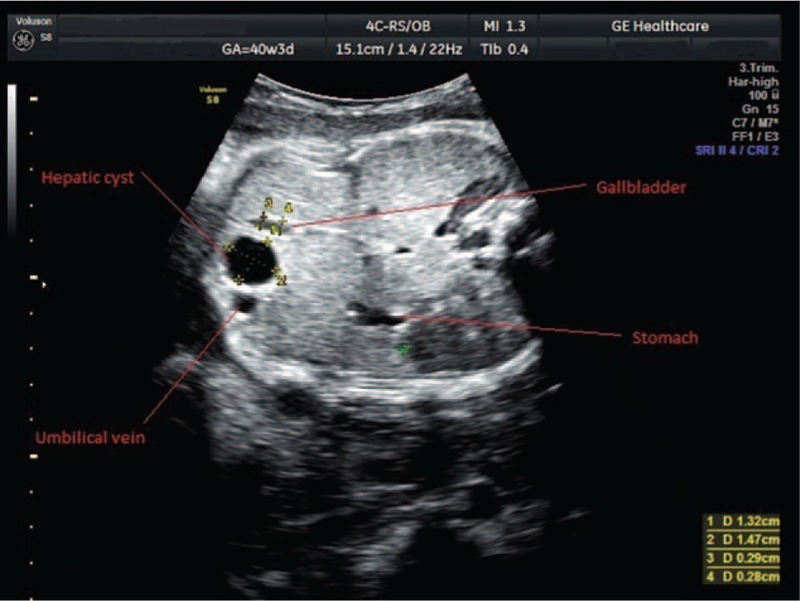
Transabdominal ultrasound of a 36-week-old fetus, showing hepatic cyst.
